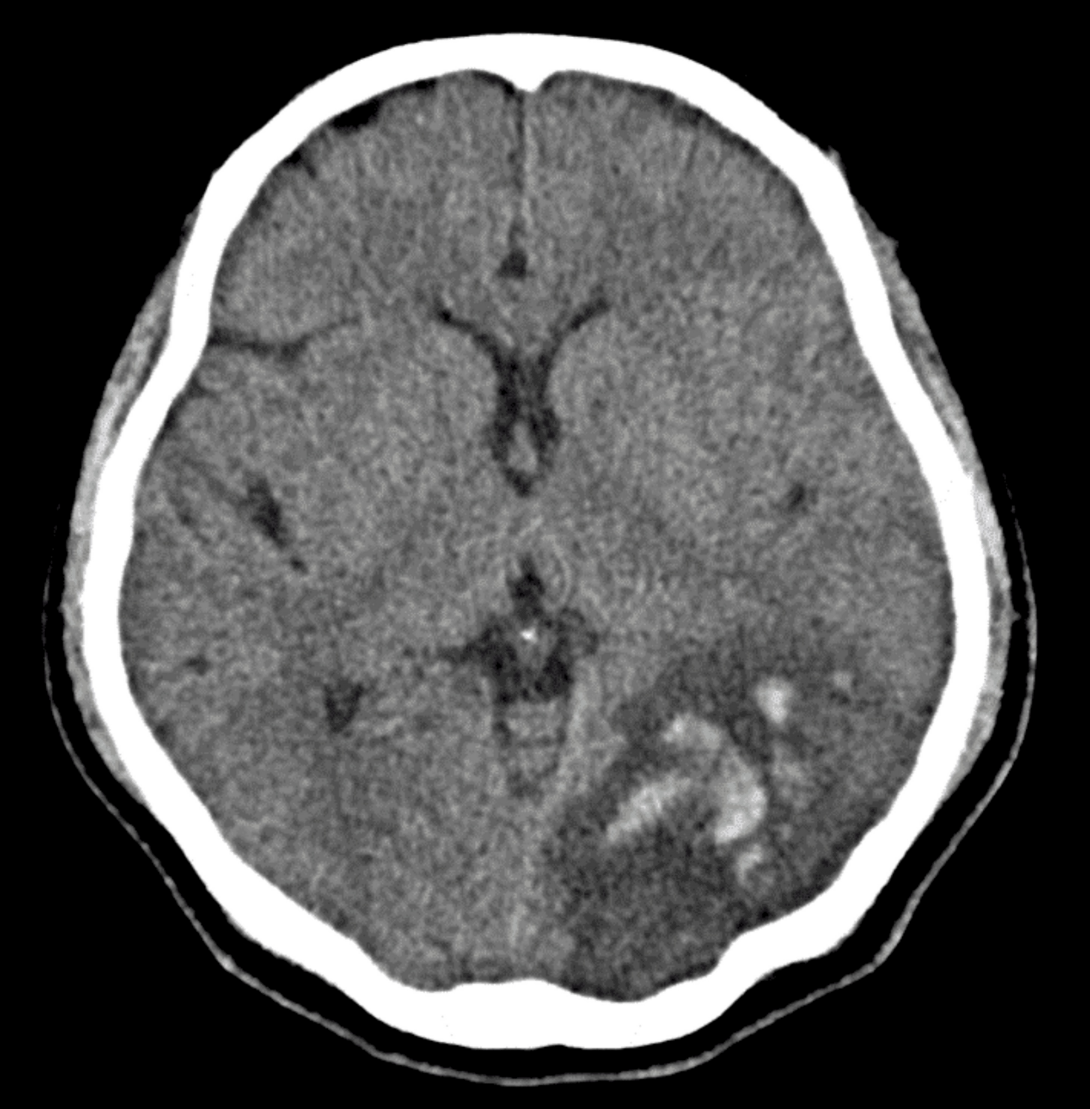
Axial non-contrast CT of the head demonstrating cerebral ischemia and foci of hemorrhage CT of the head showing a large area of parenchymal low density in the left parieto-occipital lobe. This is causing loss of grey-white matter differentiation, most likely due to subacute ischemia. There are also areas of associated hyperdensity which suggest acute parenchymal hemorrhage. CT: computed tomography
